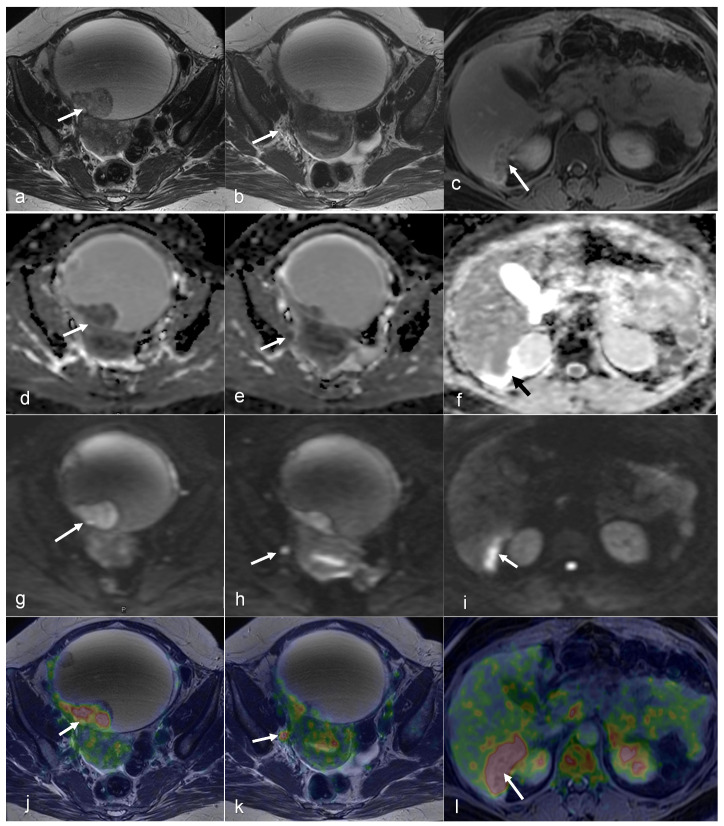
A 50-year-old female patient with peritoneal carcinomatosis secondary to ovarian clear cell carcinoma has a surgical peritoneal cancer index (PCI) score of 14 and a minimal amount of free fluid. On an axial T2-weighted image (a), a large cystic mass (hyperintense lesion) with a solid component appears slightly hypointense (arrow on (a)), shows restricted diffusion on the DWI and ADC map (arrows on (g,d)), and has high FDG uptake on fused PET/MRI (arrow in (j)). A 5 mm lymph node near the right internal iliac vessels is visibly hypointense on T2WI (arrow on (b)), shows restricted diffusion on DWI and ADC map (arrows in (h,e)), and has high FDG uptake (arrow on (k)). Notably, there is a peritoneal implant in the dorsal right liver lobe (arrows in (c,f,i,l)). (Courtesy of Elisabeth Hedlund, MD, Håkan Ahlström, MD, and Björg Jónsdóttir, MD, PhD, Uppsala University, Uppsala, Sweden).
